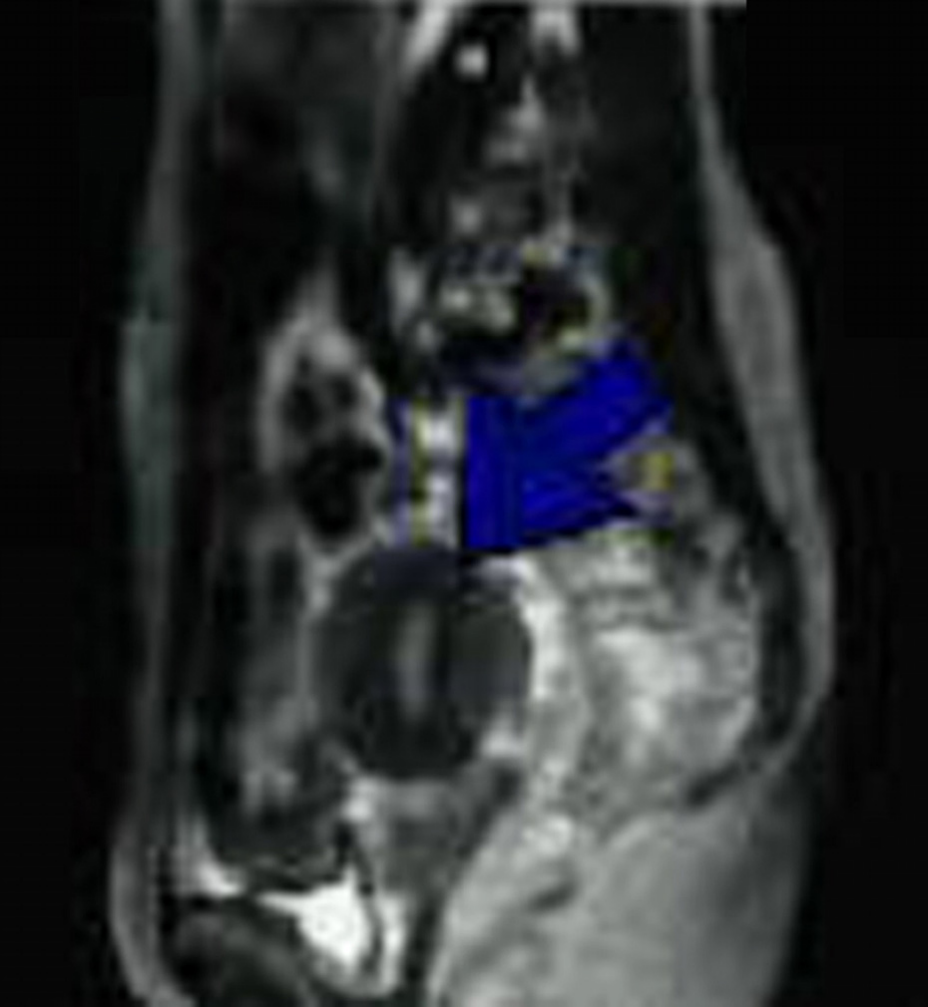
Abdominal MRI shows a 5.5 × 4 cm mass near the rectum posterior to the uterus.
